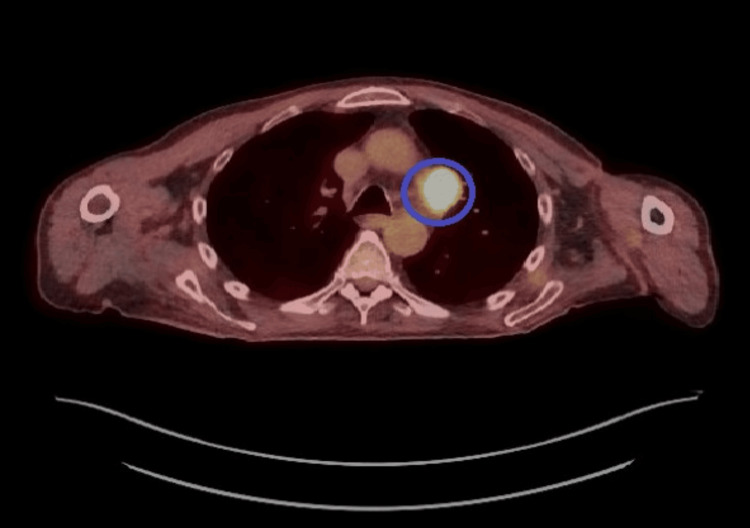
PET scan showing FDG-avid lymph node PET: positron emission tomography, FDG: fluorodeoxyglucose
